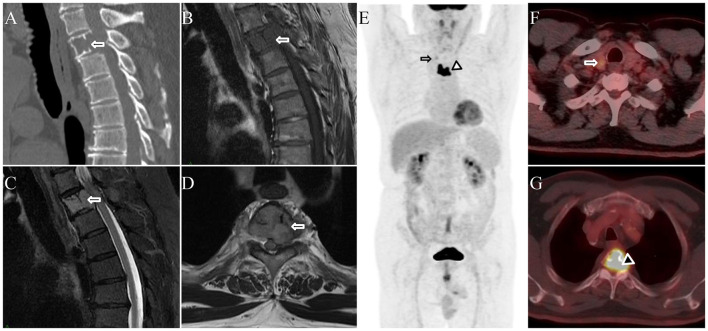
(A) CT showing osteolytic lesions involving the T3 vertebral body and bilateral pedicles, with residual bony ridges. (B) T1-weighted MRI showing hypointense signal in the T3 vertebral body. (C) Fat-suppressed T2-weighted MRI showing hyperintense signal in the same region. (D) Axial MRI demonstrating the characteristic “mini-brain”–like appearance, with narrowing of the adjacent spinal canal and left intervertebral foramen, resulting in compression of the left nerve root. (E) Maximum-intensity projection (MIP) 18F-FDG PET image showing intense uptake in the T3 vertebra (arrowhead) and mild uptake in the right thyroid lobe (arrow). (F) Axial PET/CT demonstrating a hypodense lesion with punctate calcifications in the right thyroid lobe (SUVmax 3.1). (G) Axial PET/CT showing osteolytic destruction of the T3 vertebra with posterior soft-tissue extension into the spinal canal, and markedly increased FDG uptake (SUVmax 15.2).
